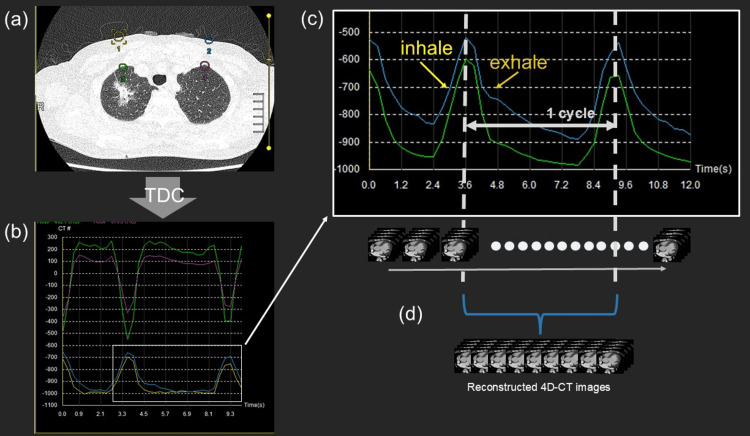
Respiratory phase-resolved image reconstruction for lung tumor motion assessment (a) Placement of ROIs on the chest wall within time-series dynamic CT data
